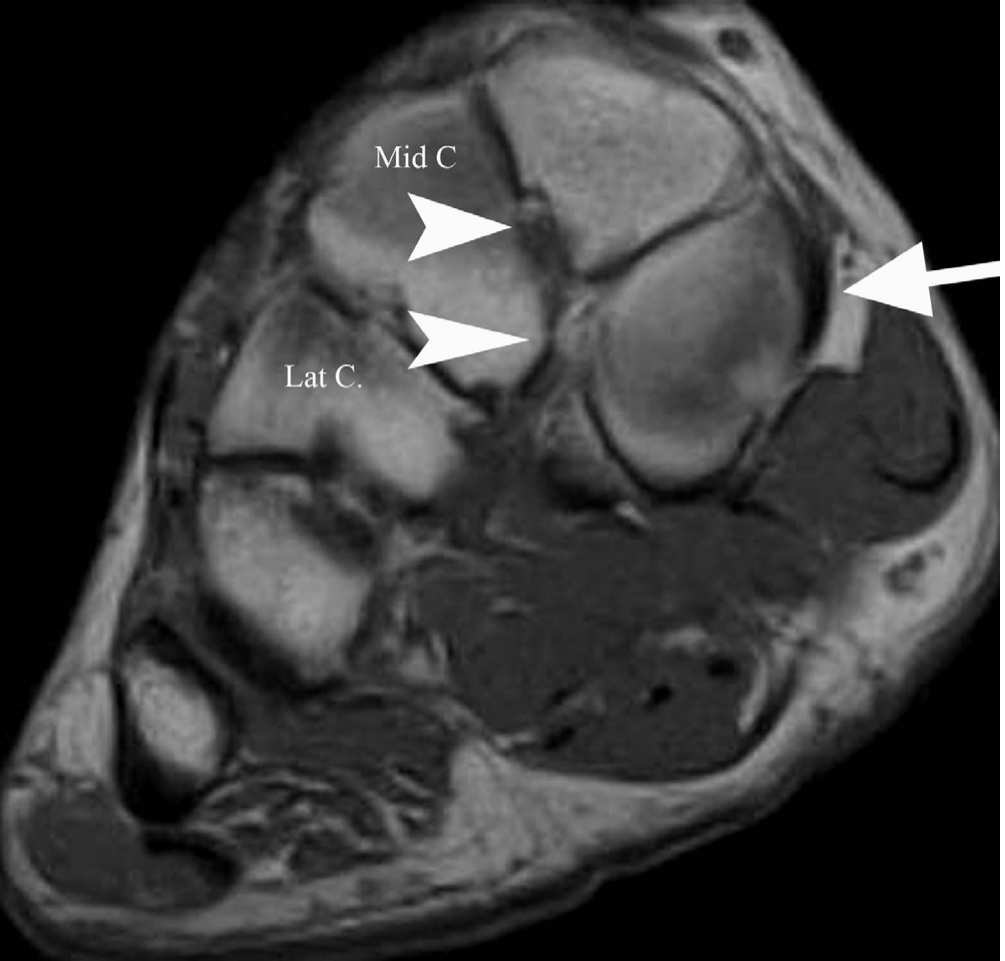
Short axis (coronal) proton density spin echo MR image. (TR/TE = 1800/12 ms) at the level of the midfoot shows a smooth, horizontally oriented, well corticated cleavage of the medial cuneiforms noted in case #2. The posterior tibial tendon inserts on the medial aspect of the plantar segment (arrow) and the dorsal and plantar bundles of the Lisfranc ligament each insert on the respective portions of the medial cuneiform (both arrowheads). Mid C = middle cuneiform; Lat C = lateral cuneiform.
